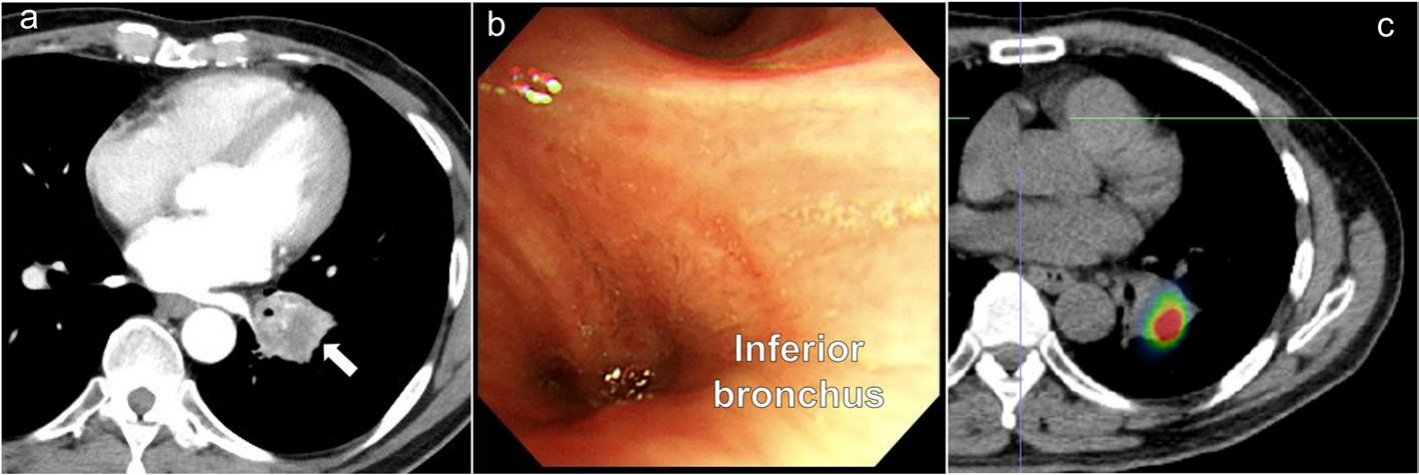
Preoperative chest computed tomography, bronchoscopic, and 18F-fluorodeoxyglucose positron emission tomography-computed tomography images. Chest computed tomography revealed a tumor in the left inferior lobe (white arrow) a Bronchoscopy showed that the left inferior bronchus had been occluded by the tumor b 18F-fluorodeoxyglucose positron emission tomography-computed tomography revealed an area of abnormal uptake, covering the tumor and the no. 11 lymph nodes c
